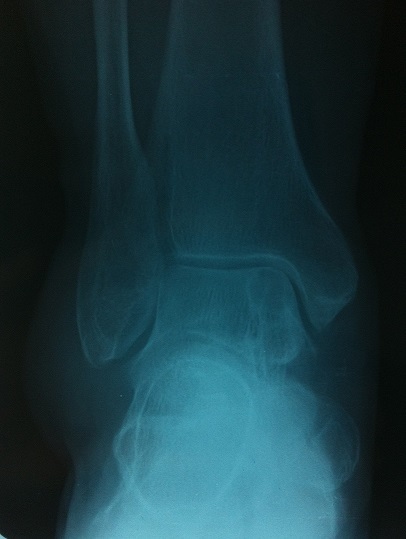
Radiographie de la cheville de face montrant une hyperdensité des parties molles
